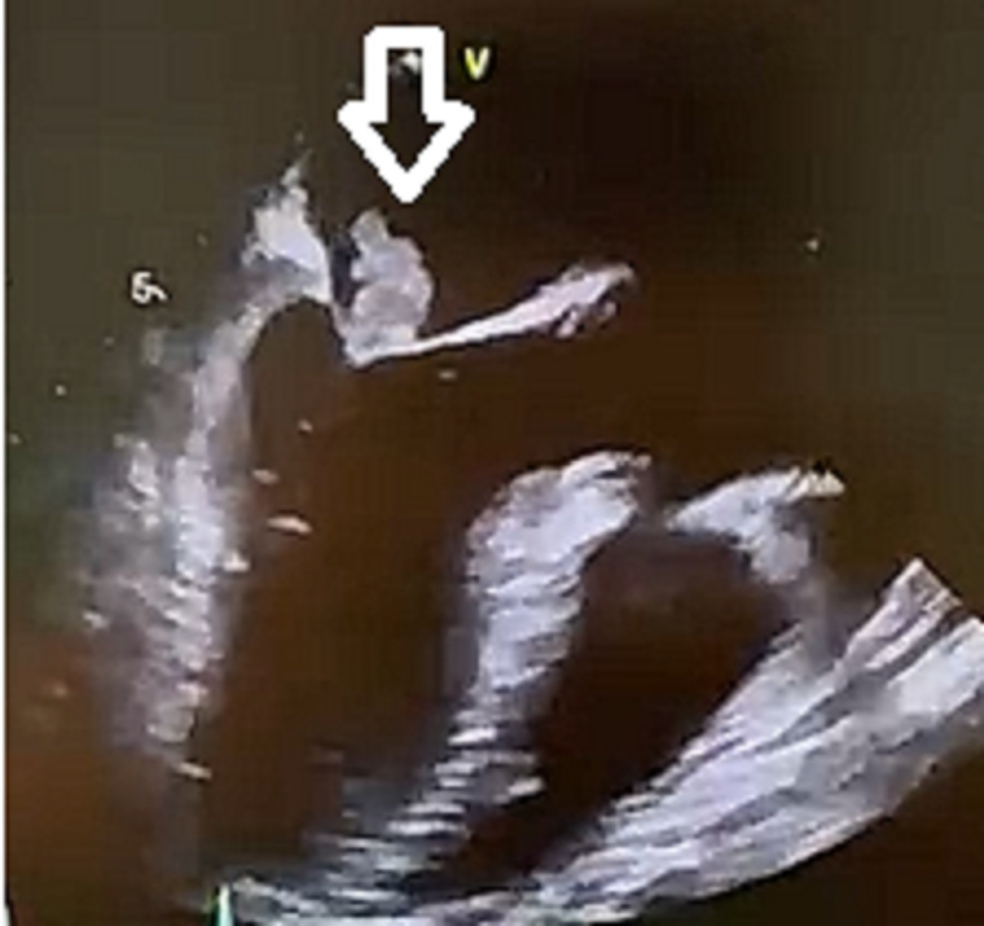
Echocardiogram Presence of mitral valve vegetation (arrow)
